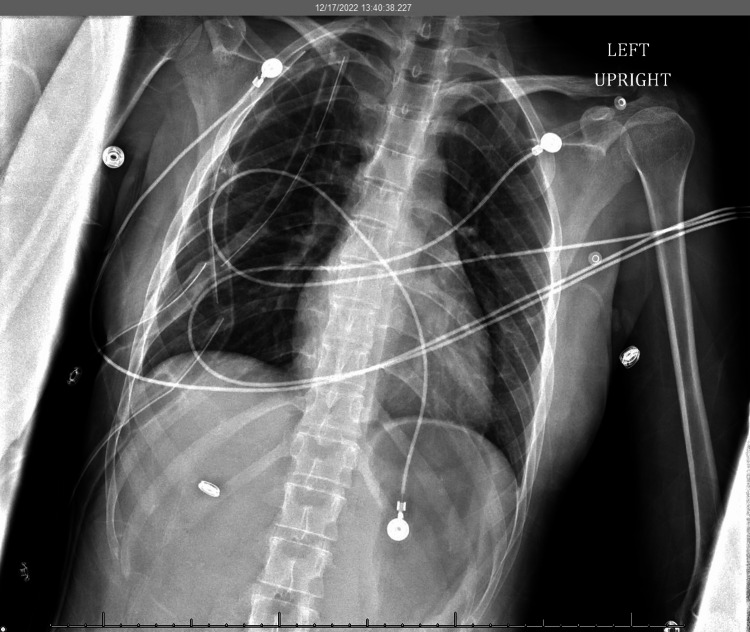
Chest x-ray done post VATS procedure Impression: Two large bore right-sided chest tubes placed. Lungs are well aerated and clear. There is no appreciable pneumothorax.
